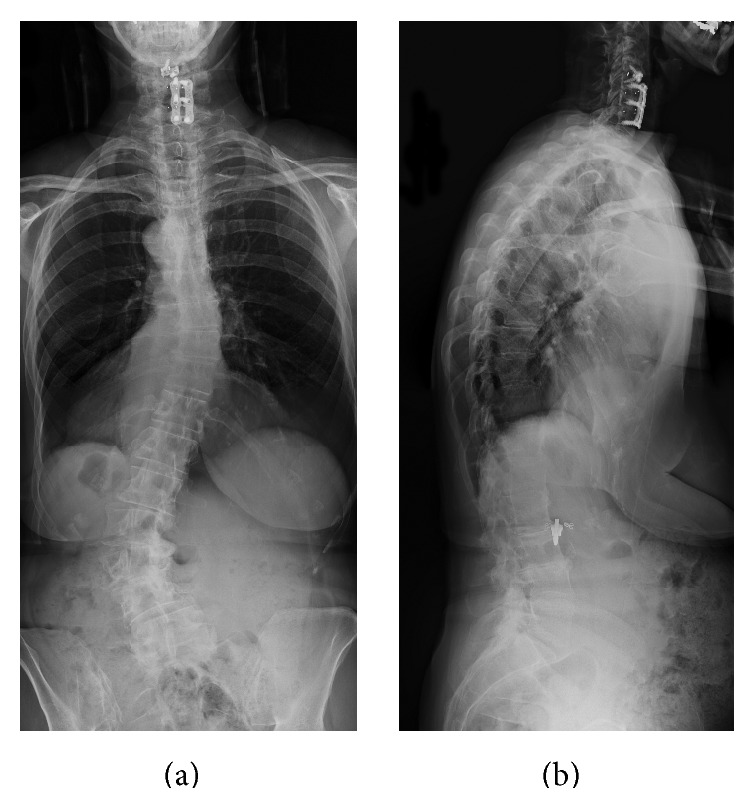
Posterior-anterior (a) and lateral (b) preoperative 3-foot scoliosis X-rays. Note the prominent curvature of the lumbar spine, which resulted in progressive symptoms.
